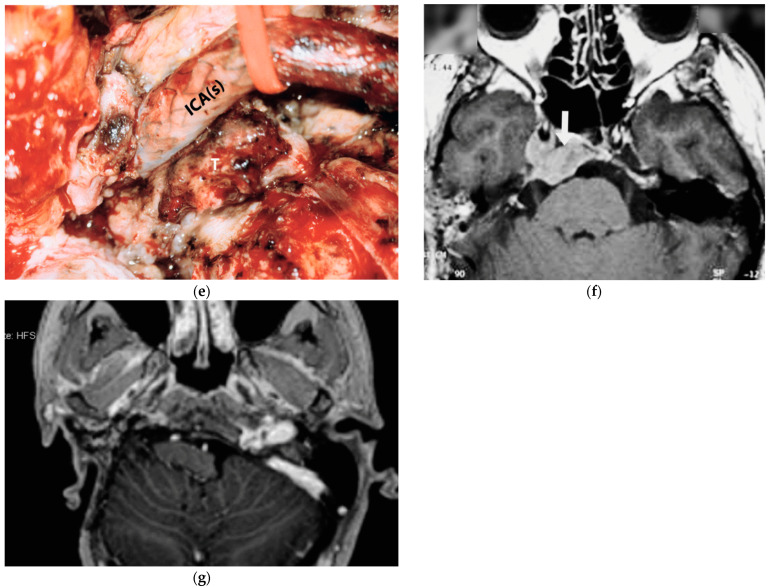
(Case 3) (a) CT scan, coronal view, of tumor extending into the craniocervical junction. (b) Axial-enhanced T1W MRI showing the tumor extension into the vertical segment of ICA. (c) Axial view, CT, after 1st stage showing residual tumor around horizontal ICA and PA. (d) X-ray screen shows ICA stenting at the level of foramen lacerum. (e) Stented ICA after removal of tumor-invaded adventitia. (f) Axial-enhanced T1W MRI showing residual tumor in the cavernous sinus. (g) Enhanced T1W MRI after 4th stage [1].
